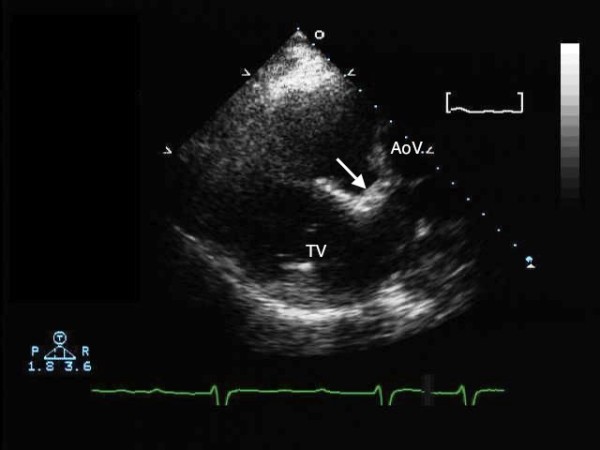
The parasternal long axis transthoracic echocardiogram shows the subaortic infundibulum (arrow), with lack of fibrous continuity between the left-sided tricuspid (TV) and aortic valves (AoV).
